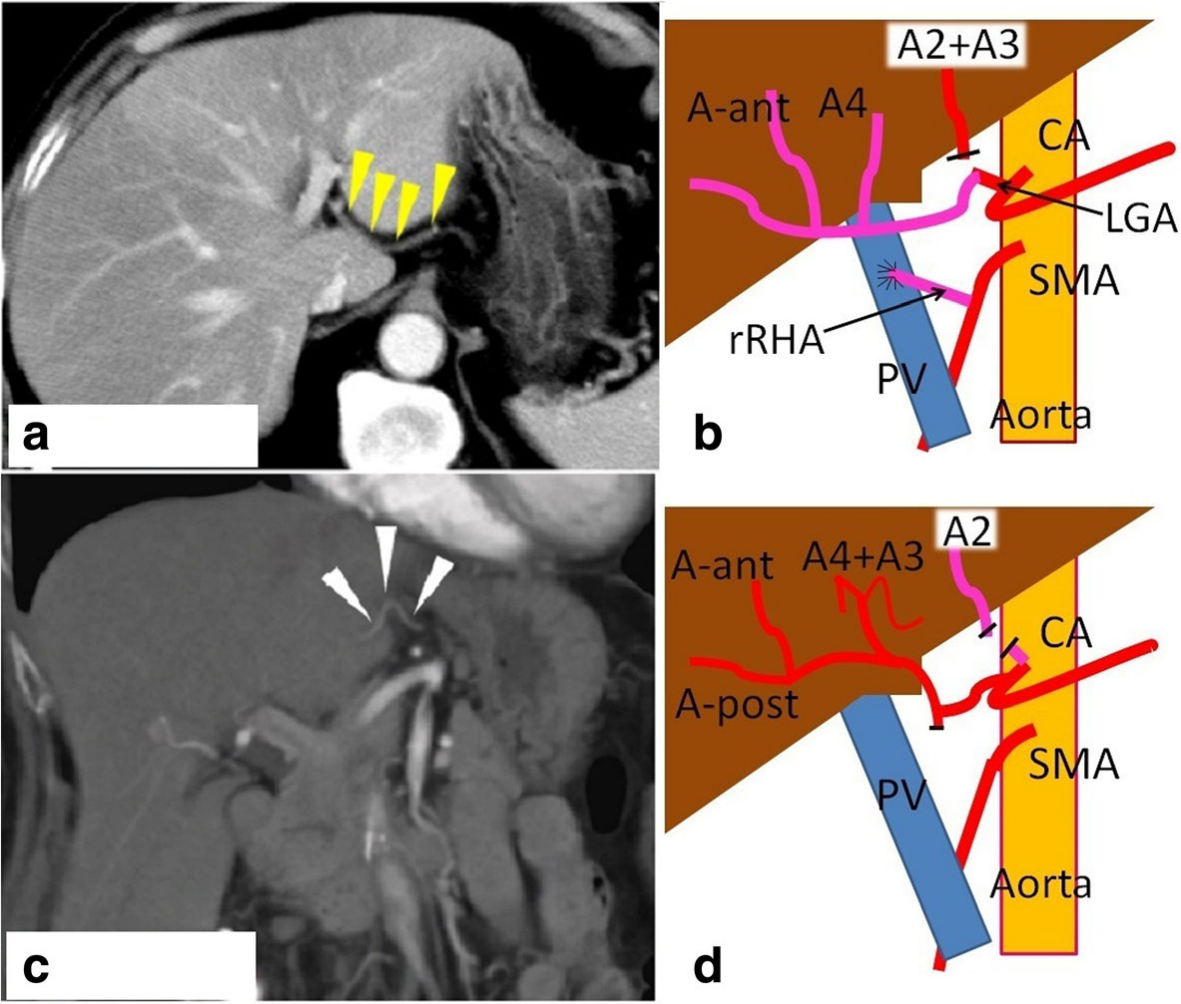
Preoperative CT and operative scheme for hepatic arteries following pancreaticoduodenectomy. a In case 1, the replaced left hepatic artery (rLHA) is segment 2/3 artery (A2 + A3) (*arrows*), which arises from the left gastric artery (LGA). b In case 1, Reconstructions of the hepatic arteries: the distal stump of the replaced right hepatic artery (rRHA) is anastomosed to the proximal stump of the LGA. The proximal stump of the rRHA is anastomosed with the left side of the portal vein (PV). The distal stump of the A2 + A3 is not reconstructed. c In case 2, the rLHA is segment 2 artery (A2) (*arrows*) only, which arises from the LGA. d In case 2, The distal stump of A2 is not reconstructed due to technical difficulties. *A-ant* anterior branch of the right hepatic artery, *A-post* posterior branch of the right hepatic artery, *A4* segment 4 artery, *A2 + A3* segment 2/3 artery, *CA* celiac axis, *LGA* left gastric artery, *rRHA* replaced right hepatic artery, *PV* portal vein, *SMA* superior mesenteric artery, *A4 + A3* segment 4/3 artery, *A2* segment 2 artery
